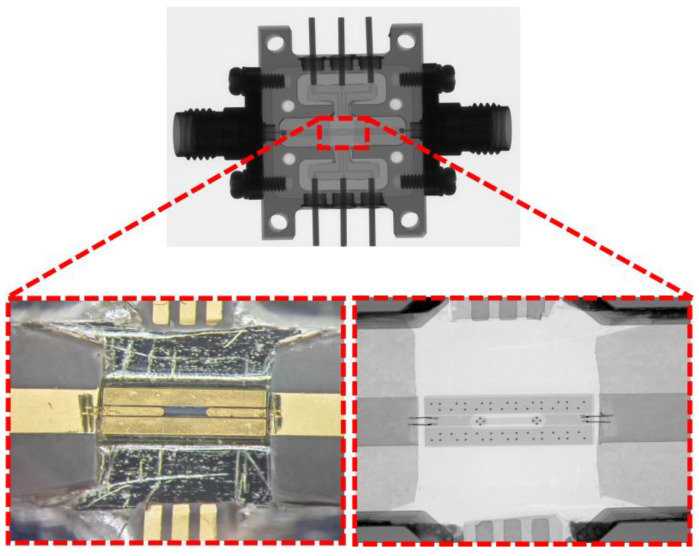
X-ray image of the test structure after mounting, with the RF TGV connected CPW structure highlighted within the red dashed box.
